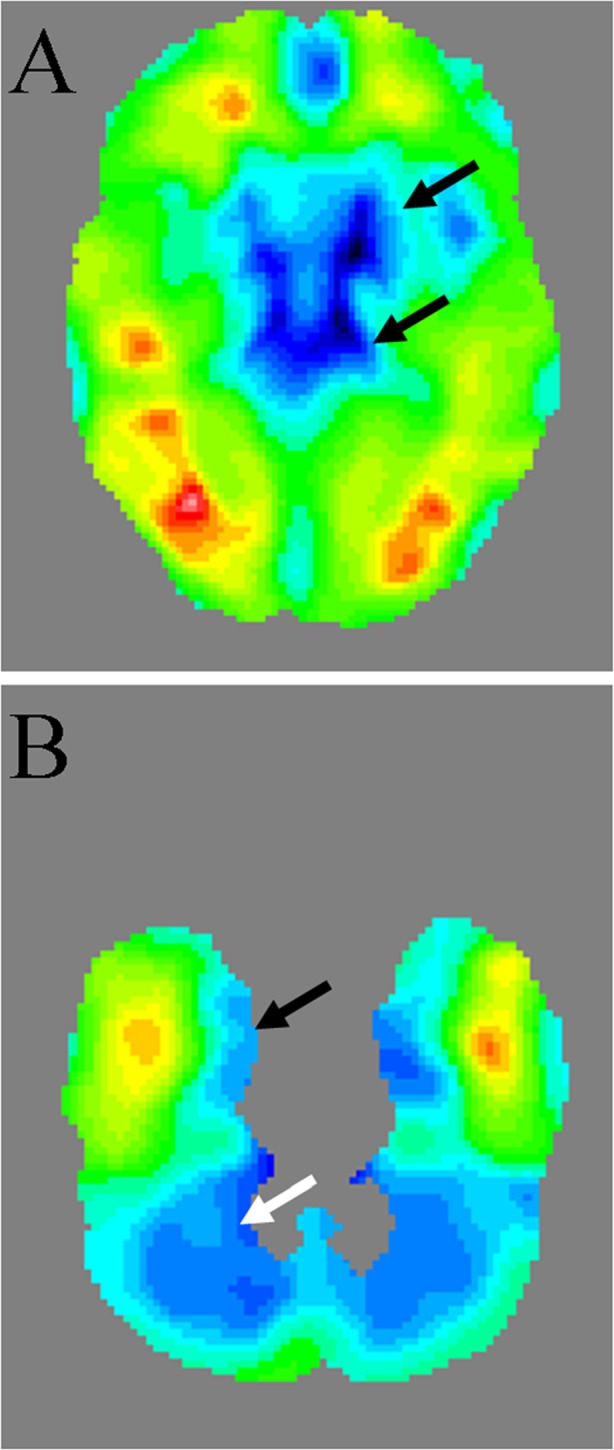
Baseline (before starting KD) 18FDG PET, distribution of standard deviations from the template. A decreased glucose uptake in the basal ganglia– arrows (SD– in the lenticular nucleus– right 3.1, left 3.0, in the thalamus– right 5.3, left 6.5). B decreased glucose uptake in the mesial temporal lobes– black arrow and cerebellum– white arrow (SD- in the mesial temporal lobe 18FDG PET– right 3.5, left 5.0, in the cerebellum– right 4.7, left 4.9). SD– standard deviation
